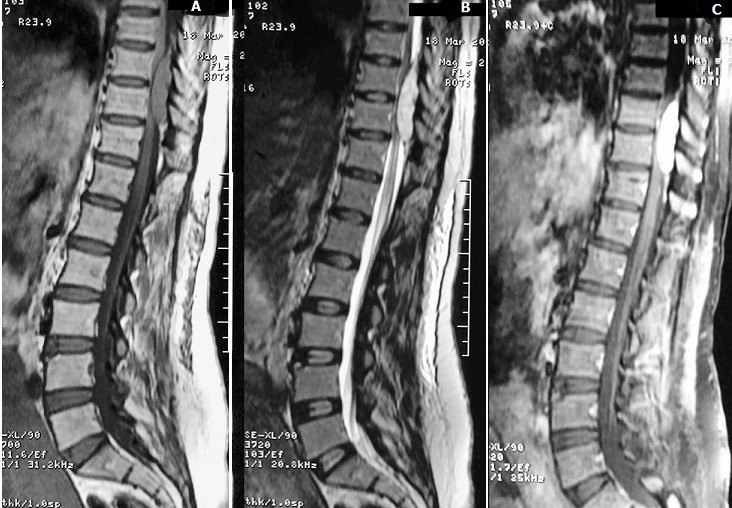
Sagittal unenhanced T1 weighted MR images: A) shows a homogeneous well-defined, ovoid epidural mass with isointense signal relative to the spinal cord, with a «wafting silk» sign. Sagittal T2-weighted images; B) shows a heterogeneous hyperintense epidural mass. Sagittal contrast-enhanced T1-weighted images; C) shows homogeneous intense enhancement of the mass
